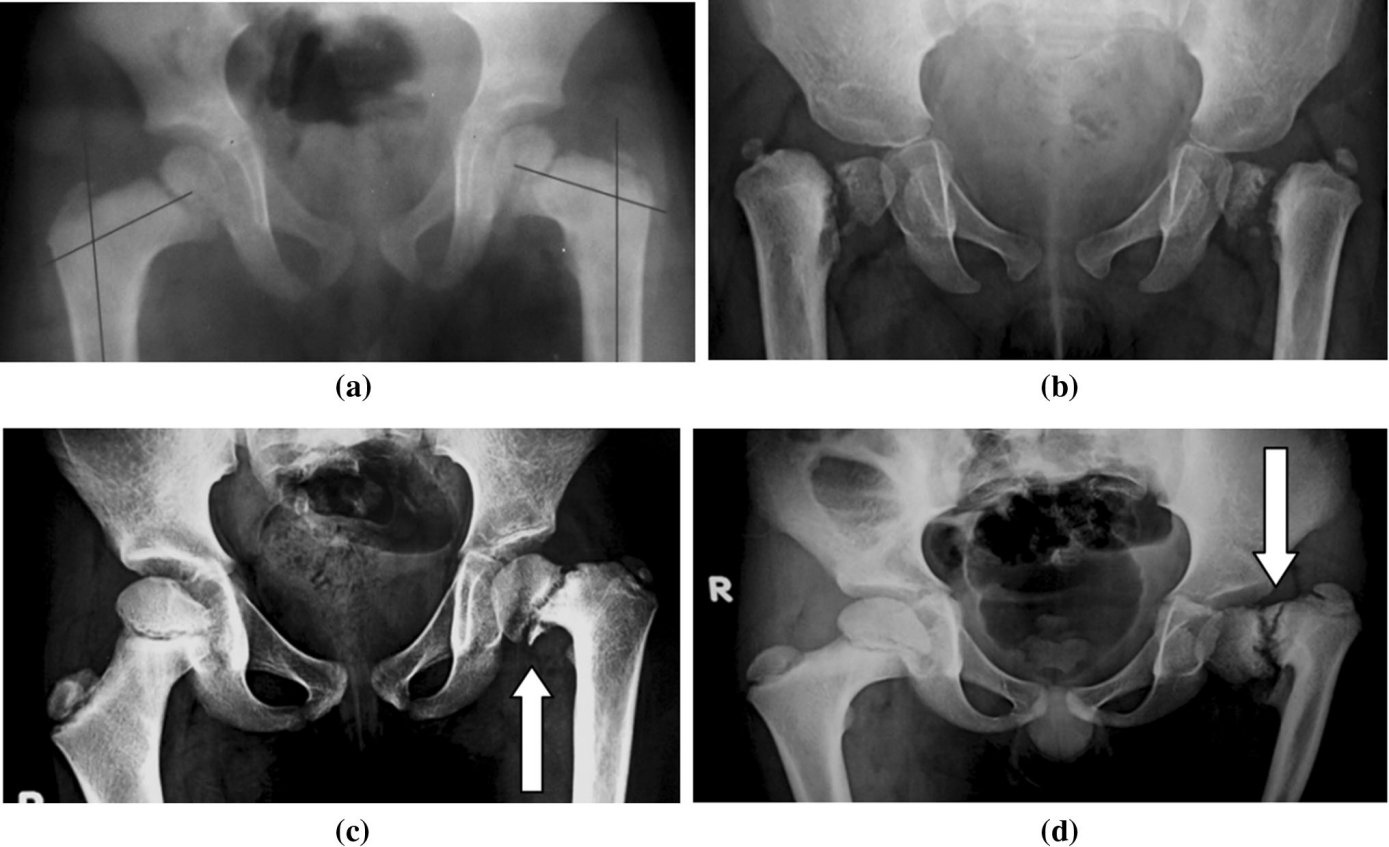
Standard AP radiographs of the pelvis and both hip joints showing different etiologies and severities of coxa vara. a Bilateral coxa vara following healed rickets. b Bilateral developmental coxa vara with a medial femoral neck defect and a vertically oriented physeal plate. c Typical unilateral coxa vara with a medial (lower) femoral neck defect. d Atypical unilateral coxa vara with a lateral (upper) femoral neck defect
